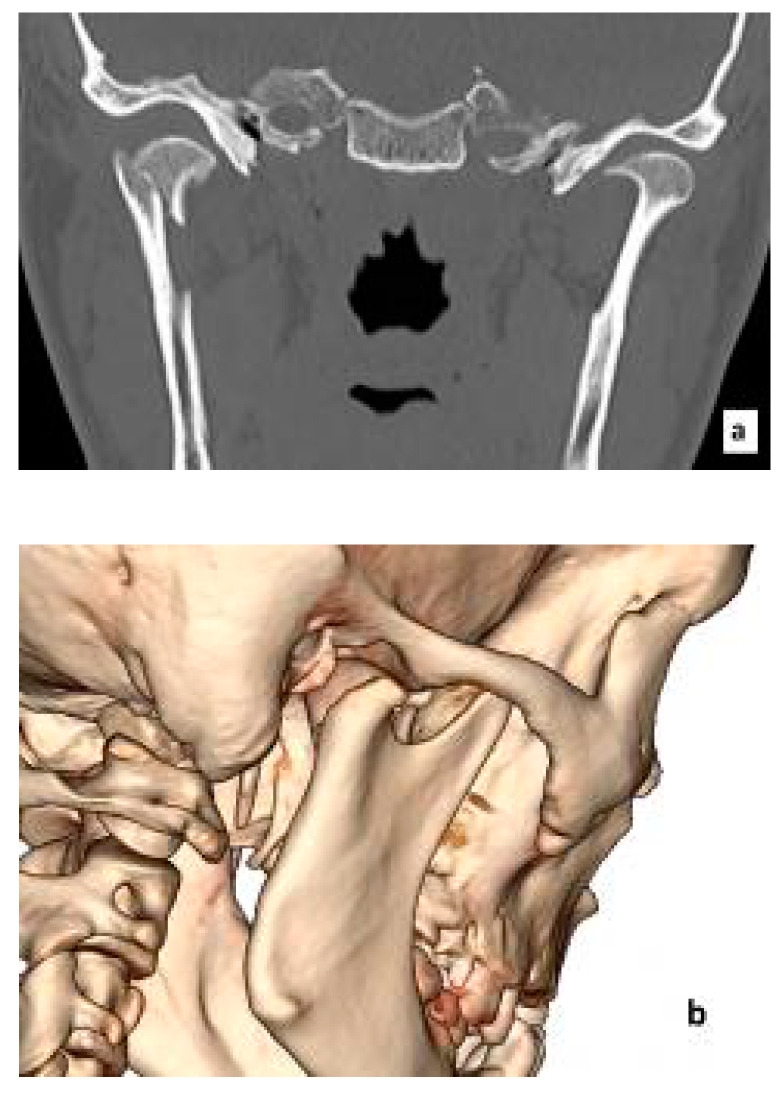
Fracture of the right mandibular head. Computed tomography: frontal scan (a) and 3D reconstruction (b).
